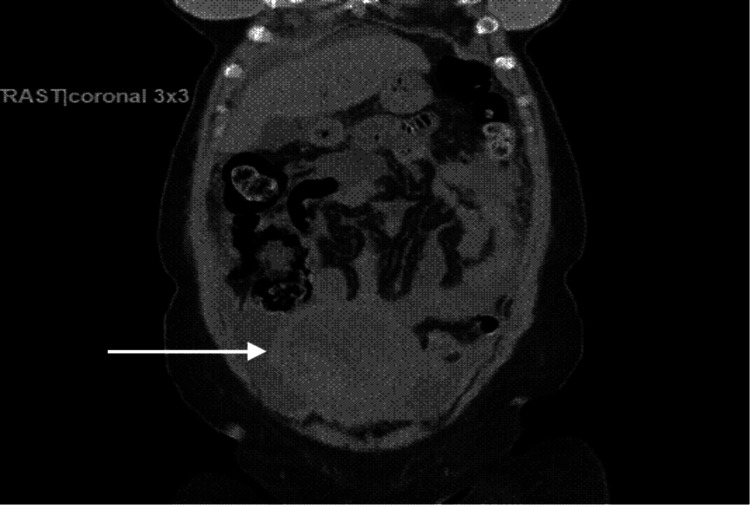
Coronal section of abdomen computed tomography scan without enhanced contrast shows an enlarged heterogeneous uterus with a small to moderate amount of ascites.
